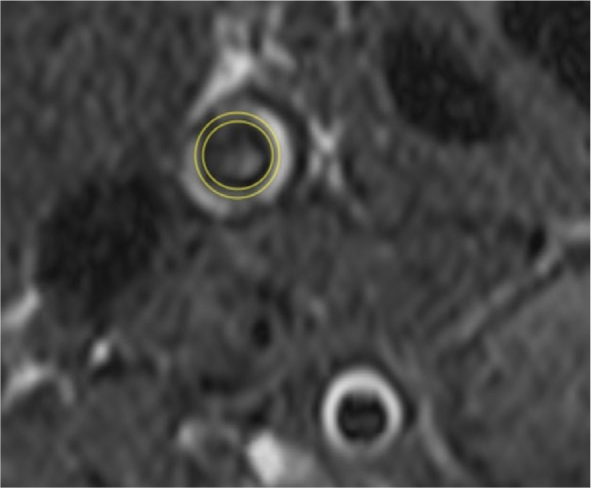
The MR imaging of the common right carotid artery. T1-weighted dark blood fast-spin echo pulse sequence demonstrated adequate soft tissue contrast. The intimamedia thickness of the vessel wall is outlined.
